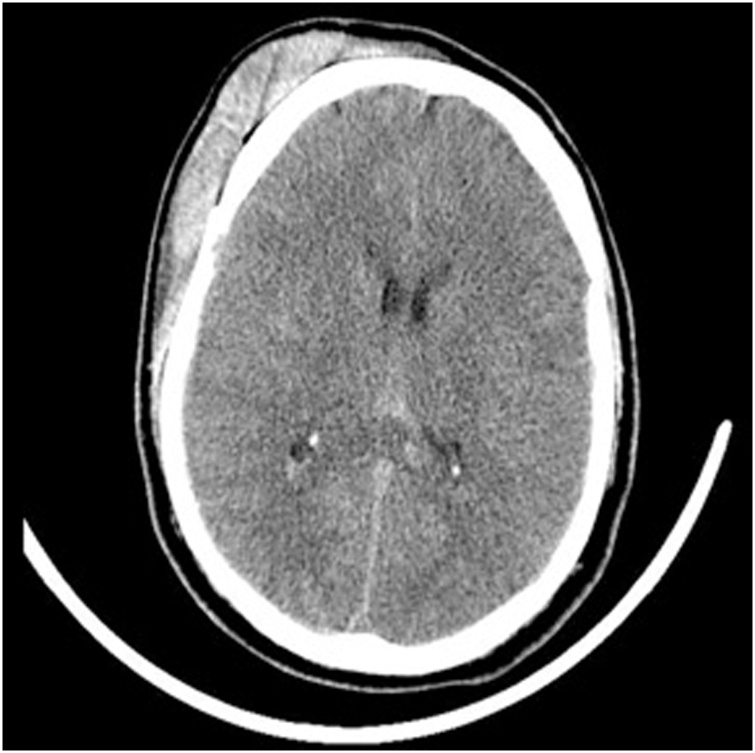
CT scan of head on admission showing subdural, subarachnoid, intraperenchymal hemorrhages and small areas of brainstem hemorrhage.
